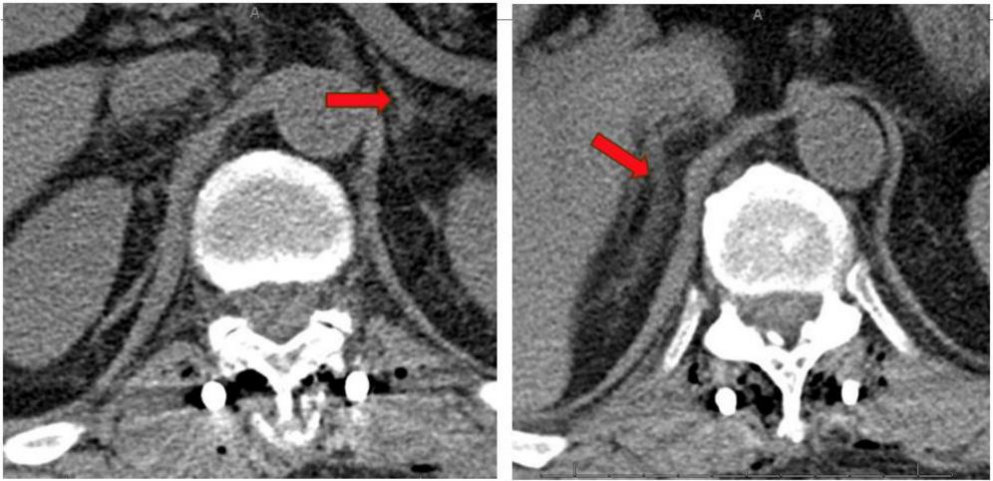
CT of thoracic/lumbar spine revealing normal-appearing left and right adrenal glands after the fall.
